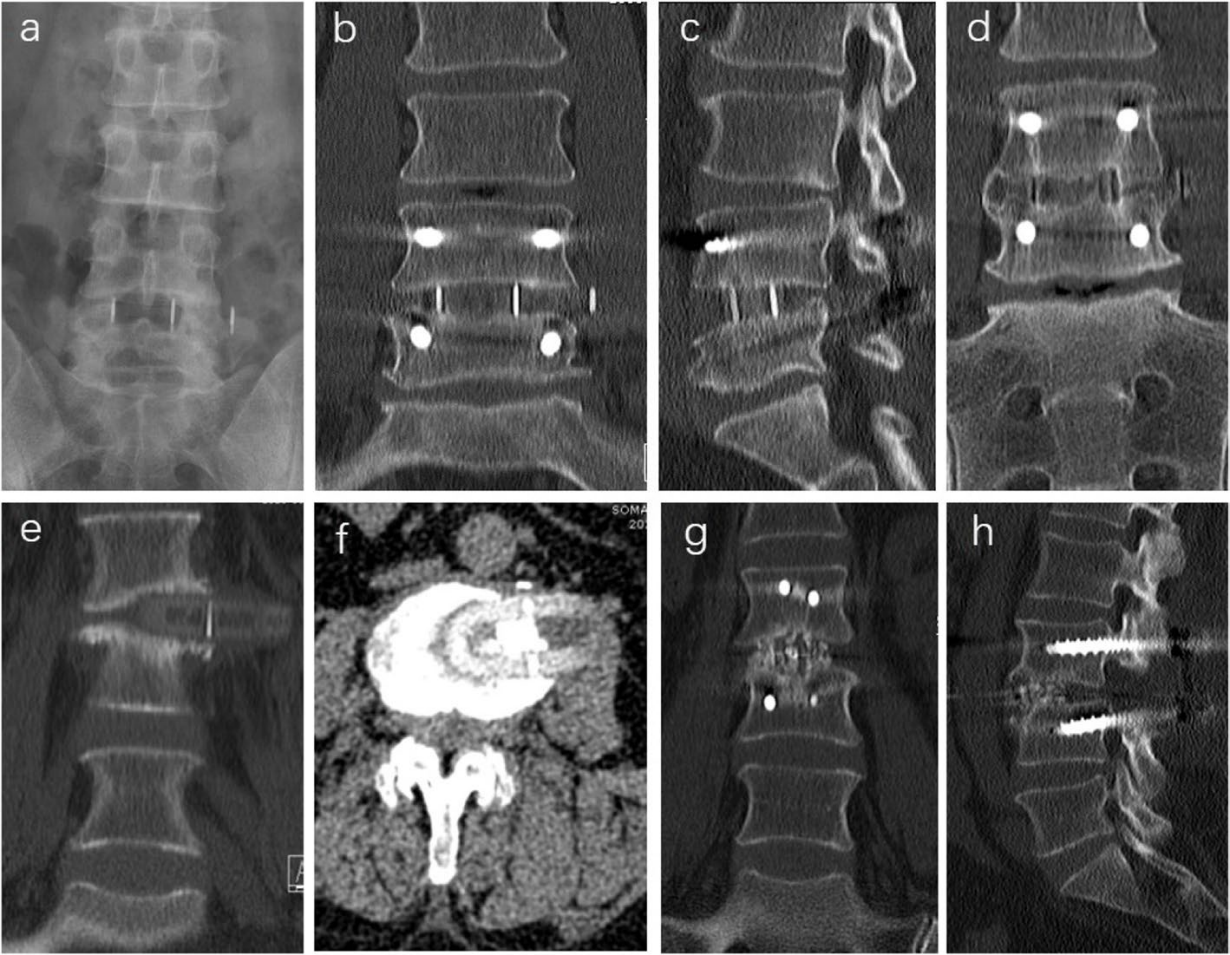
A case of cage displacement after LLIF a-d. a Cage displacement can be seen in anterior X-ray. b, c Coronal and sagittal CT of lumbar spine after revision. d Coronal CTshowed bony fusion at two years after operation. A case of cage prolapse after LLIF e-h. e, f Coronal and axial CT showed cage prolapse at three months after OLIF. g, h Coronal and sagittal CT after revision
